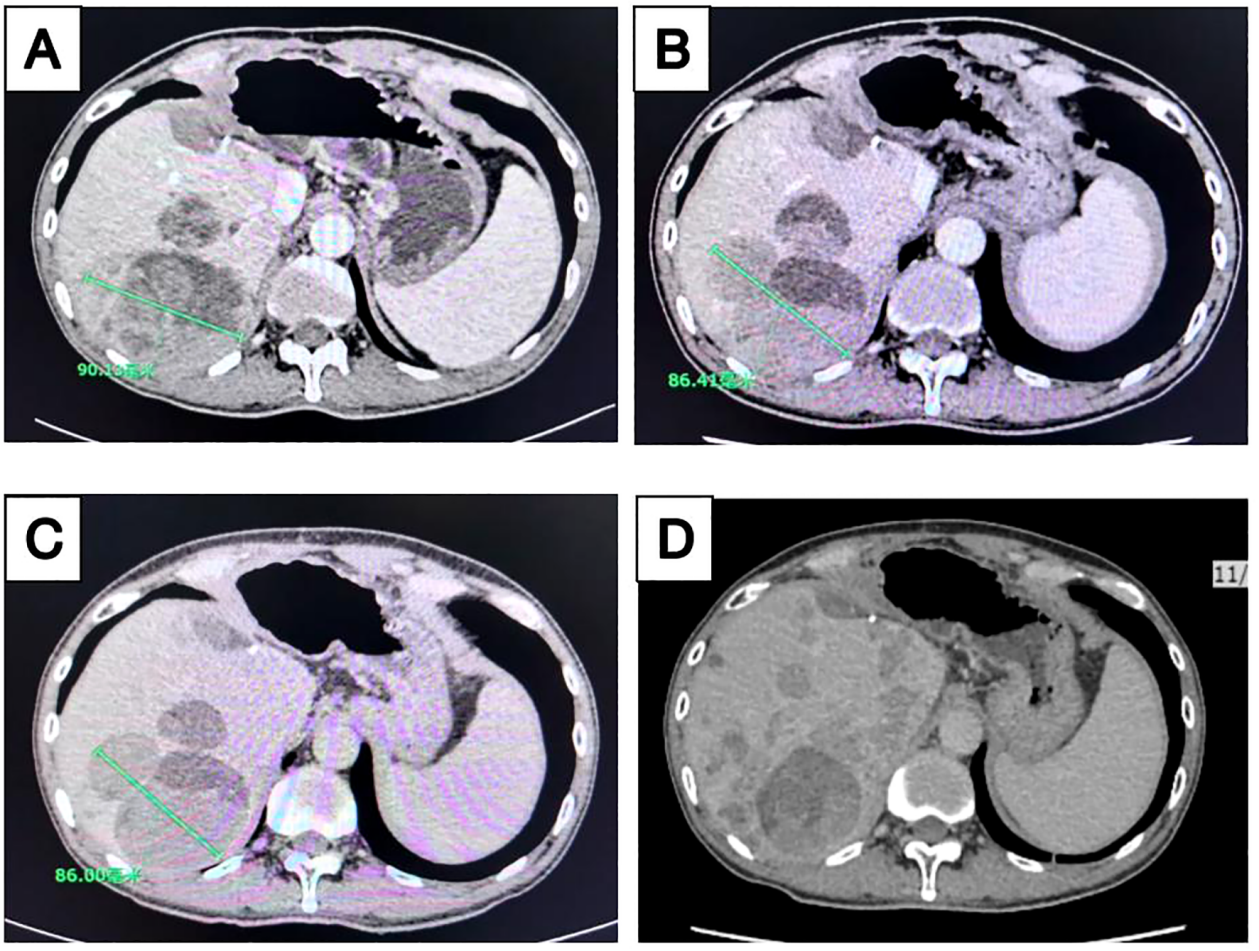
Longitudinal imaging assessment of liver lesions following treatment. Contrast-enhanced computed tomography (CT) scans of the liver acquired at multiple time points during treatment: (A) April 2022, (B) June 2022, (C) August 2022, and (D) November 2022.
